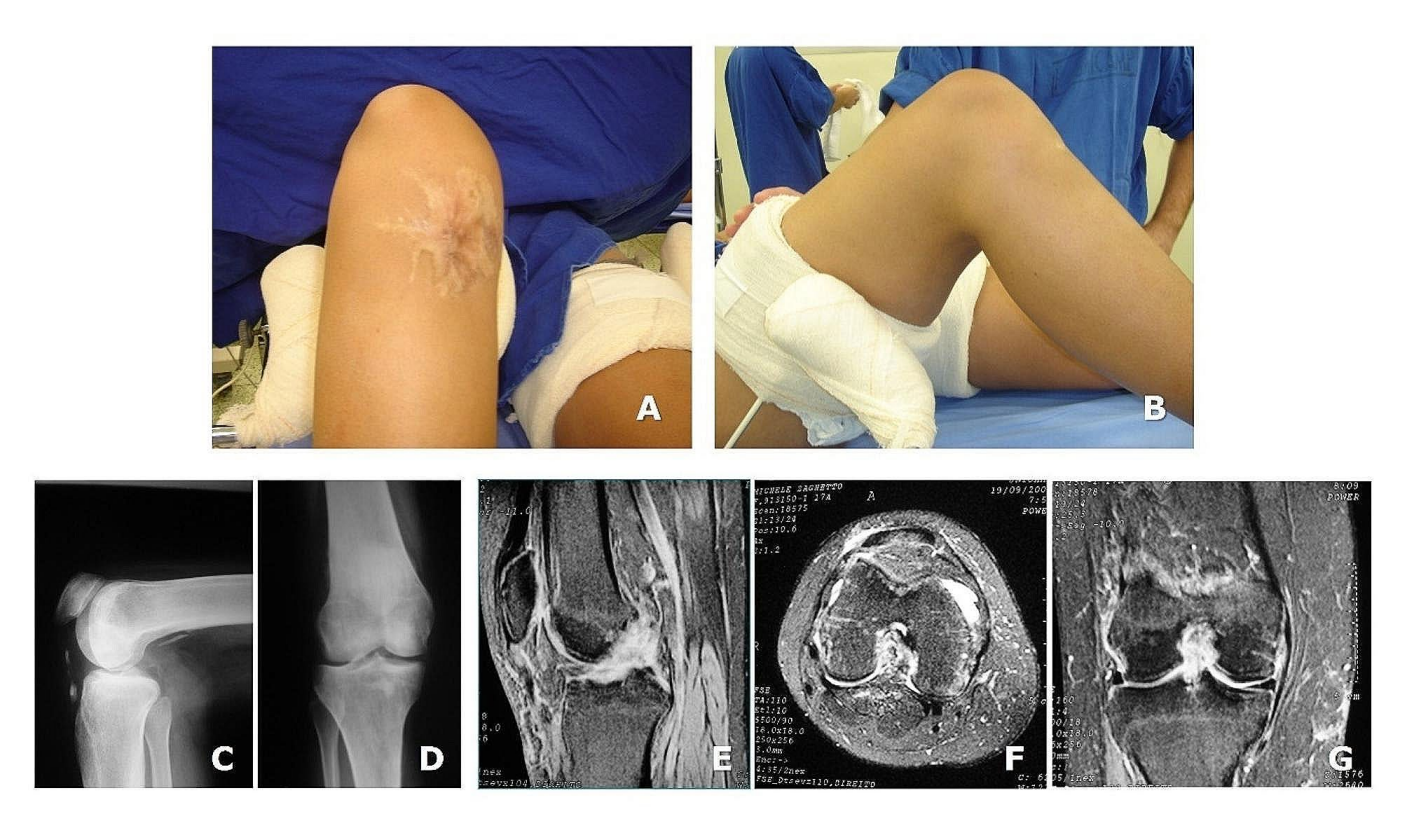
One year after the accident, on physical examination a well-healed scar (A) and posterior sag sign (B), indicating PCL insufficiency, Panels C to G: Imaging appearance (C and D: plain radiographs); E to G: sagittal, axial, and coronal MRI scans. They demonstrate the chronic patellar tendon rupture and the tear of both the anterior and posterior cruciate ligaments, a chondral Injury in the medial femoral condyle, a grade-II medial collateral ligament injury, and a minor medial meniscus injury, with no ligament injury of the lateral knee compartment, as also confirmed during the surgical procedure
